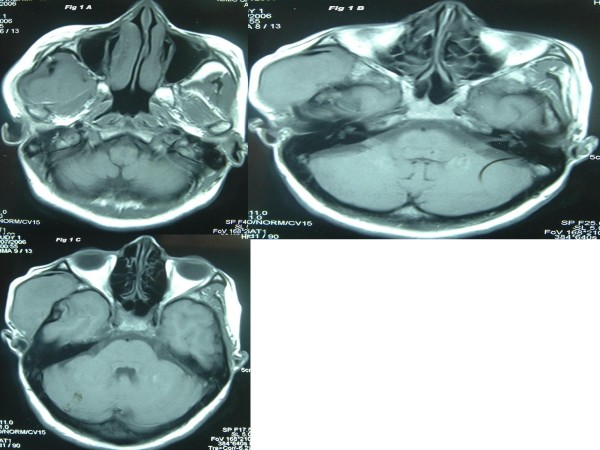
A-C: MRI (axial sections) showing a big hypo-isointense mass in the right temporal and infratemporal fossa on T1 weighted images.
